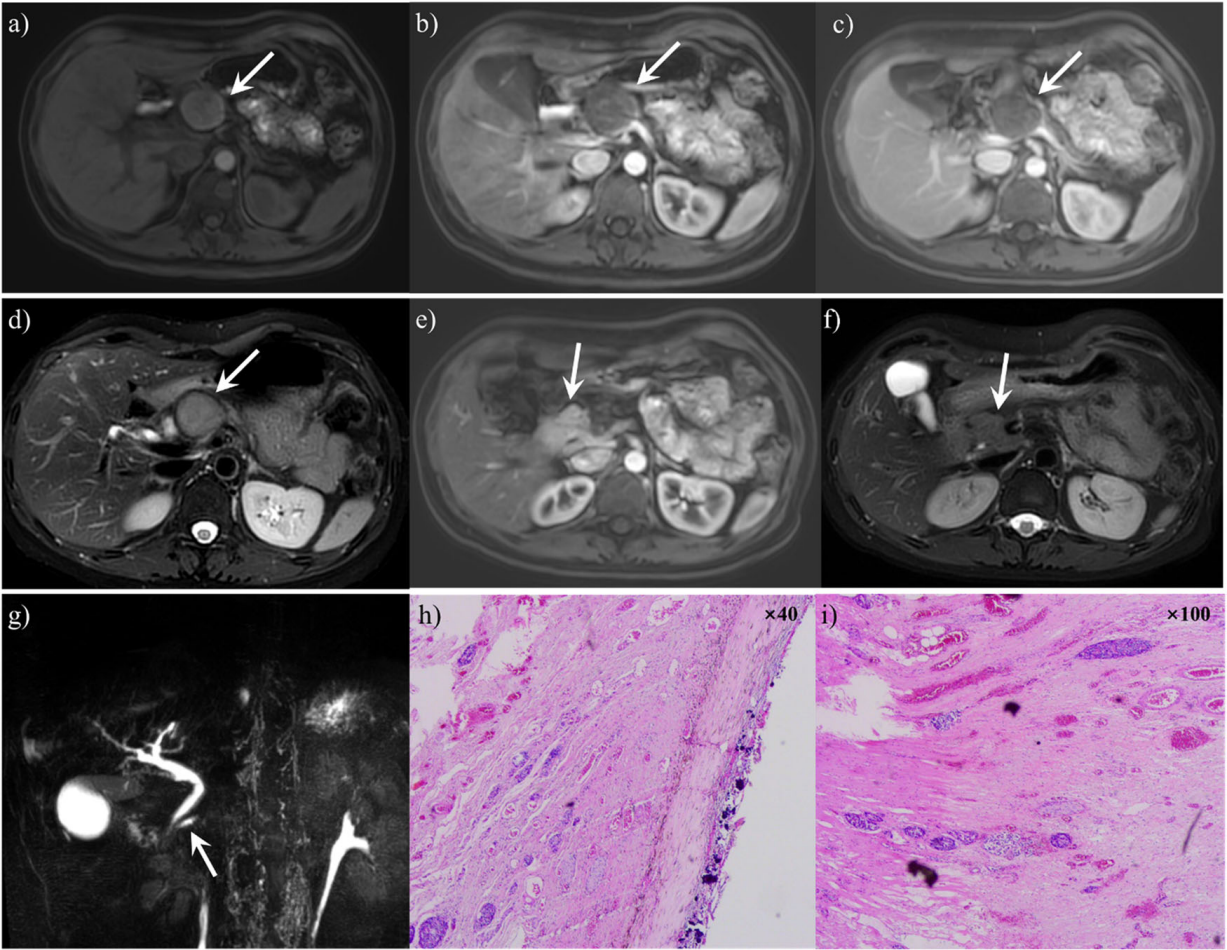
A 42-year-old woman presented with dorsal pancreatic agenesis and a pancreatic pseudocyst. a Axial T1-weighted fat-suppressed MR image revealed a well-defined mass (arrow) with slightly hyperintensity and peripheral hyperintensity. Arterial (b) and venous (c) phase MR images showed that the tumor had no significant enhancement. The tumor (arrow) showed a slightly hyperintense signal on axial T2-weighted MR image (d). Arterial phase MR image (e) and T2-weighted MR image (f) showed that only the head of the pancreas was visible (arrow). Coronal Magnetic Resonance Cholangiopancreatography (MRCP) (g) showed that the main pancreatic duct (arrow) was short and the pancreatic duct in the body and tail of the pancreas was not visualized. h, i Pathology confirmed a pancreatic pseudocyst, though post-tumor change after complete regression could not be excluded
